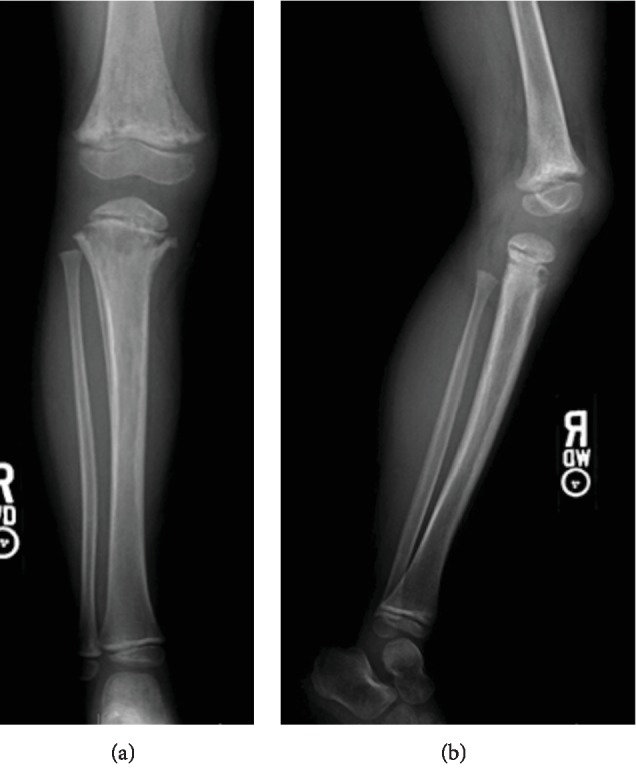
Anteroposterior (a) and lateral (b) plain radiographs of the patient two months after IO cannulation revealing progression of lytic processes in the distal femur and proximal tibia concerning for osteomyelitis.
